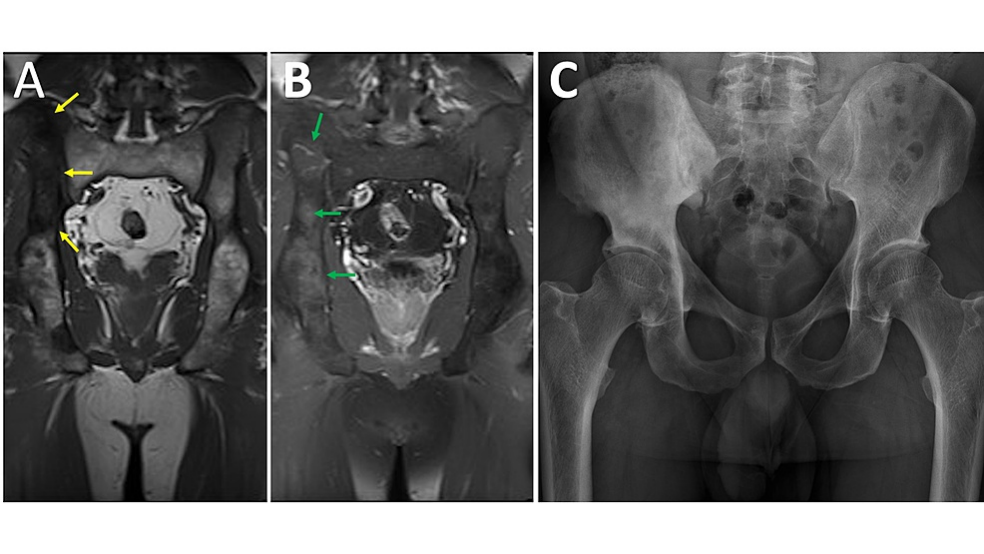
Paget MRI coronal T1 of the pelvis (14A) and coronal T1 post-contrast fat saturation (FS) of the pelvis (14B) demonstrates T1 hypointensity (yellow arrows) in the cranial aspect of the right iliac wing with diffuse speckled contrast enhancement (green arrows) relative to the contralateral osseous structures. A frontal radiograph of the pelvis (14C) demonstrates hypertrophic right side iliac wing and innominate bone with a slightly expanded appearance and thickened trabecula. No evidence of periarticular soft tissue calcification, destructive or blastic lesion, or other abnormality.
